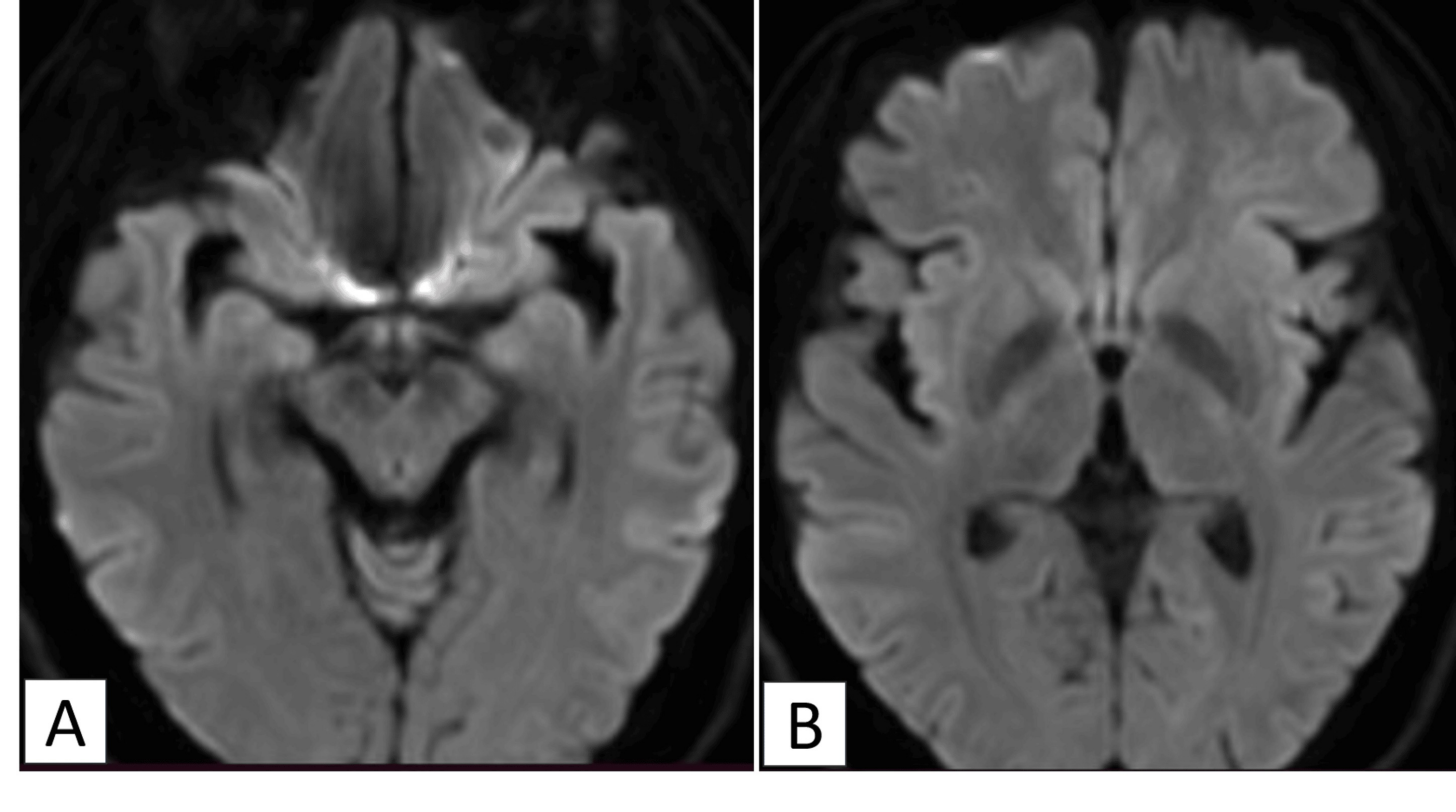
Case 1: Follow-up axial diffusion-weighted images (DWI) on day 214 The image demonstrates complete resolution of the previously noted hyperintense lesions in the (A) right lateral amygdala and the (B) left thalamus.
